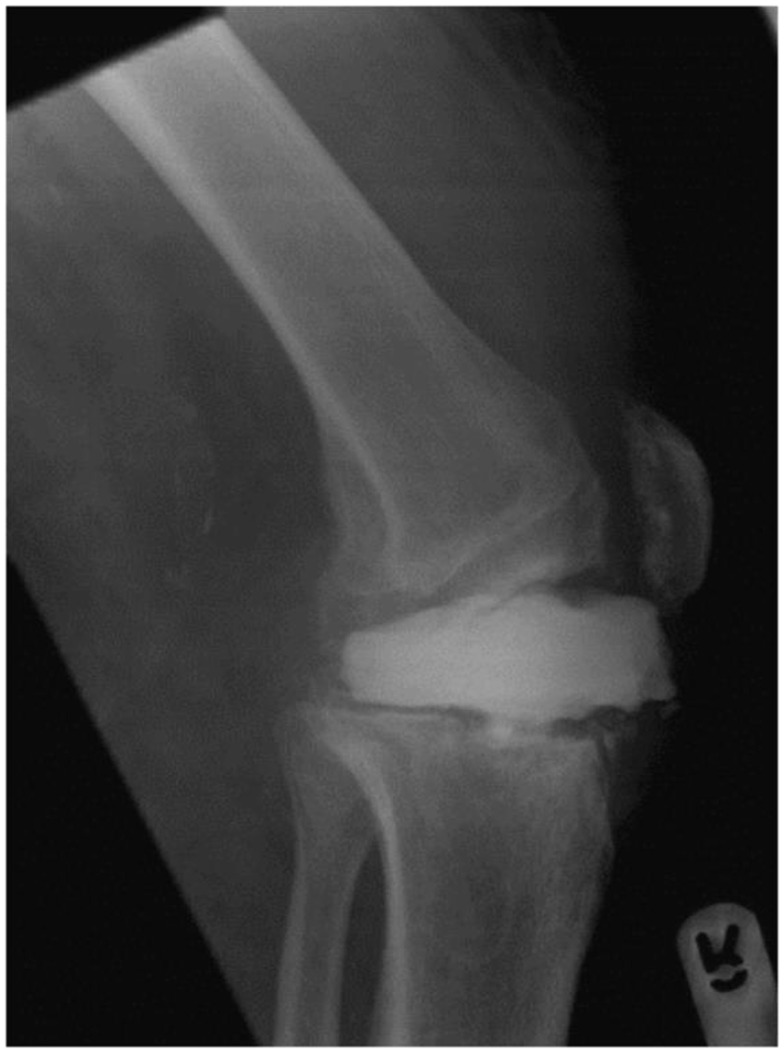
Partial rupture of the patella tendon in Case 2 demonstrated by the lateral radiographs of the knee showing anterior displacement of the static cement spacer blocks. Note that the block was not keyed in.
